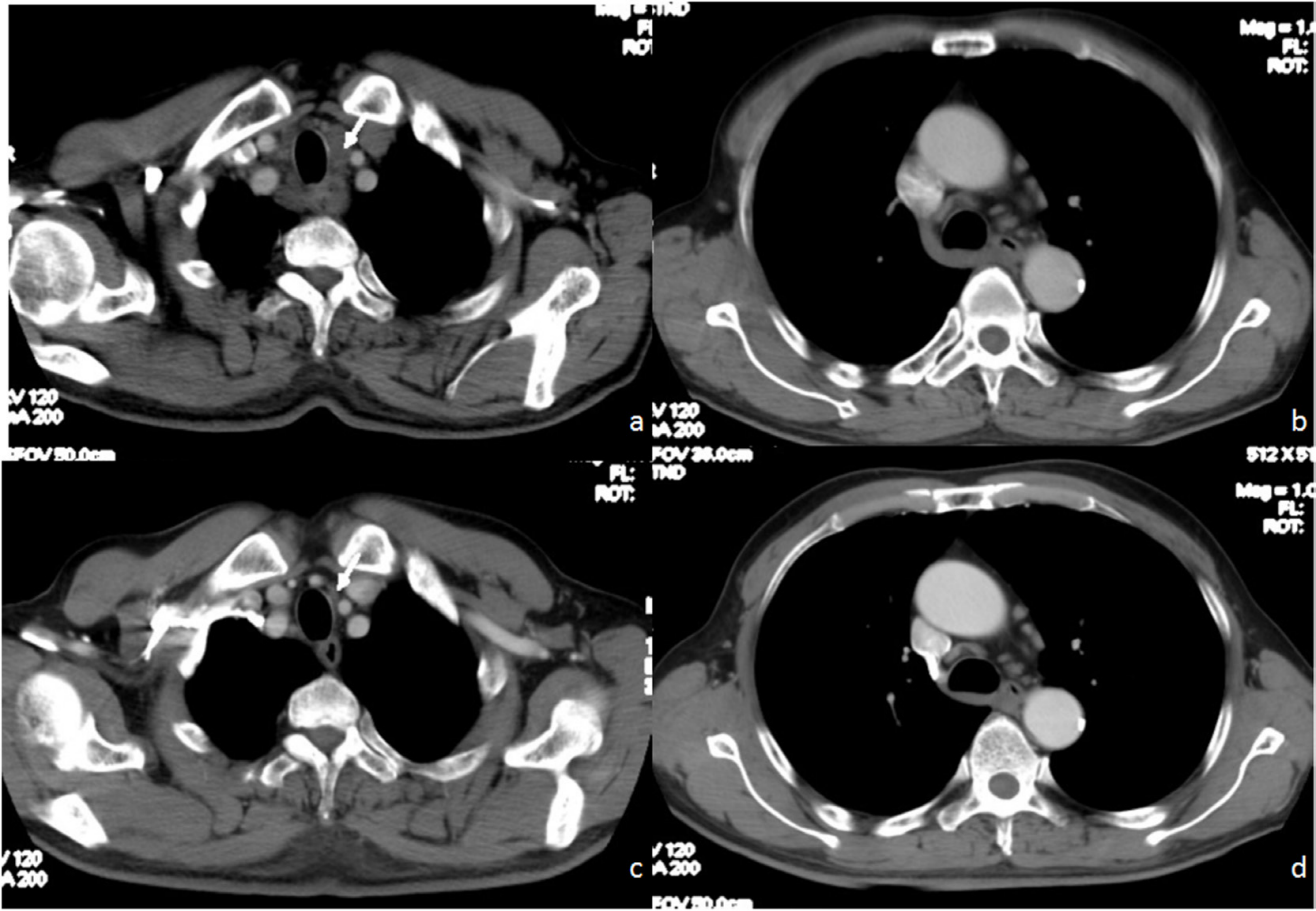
66 year-old man with poor prognosis of esophageal squamous cell carcinoma Pre-neoadjuvant chemotherapy CT (a, b) showed MaxLN (arrow): 24x13 mm, the number of LN was 10. Post-neoadjuvant chemotherapy CT (c, d) showed MaxLN (arrow):10x6mm, the number of LN was 9. OS=7.3 months, DFS=5.6 months.
